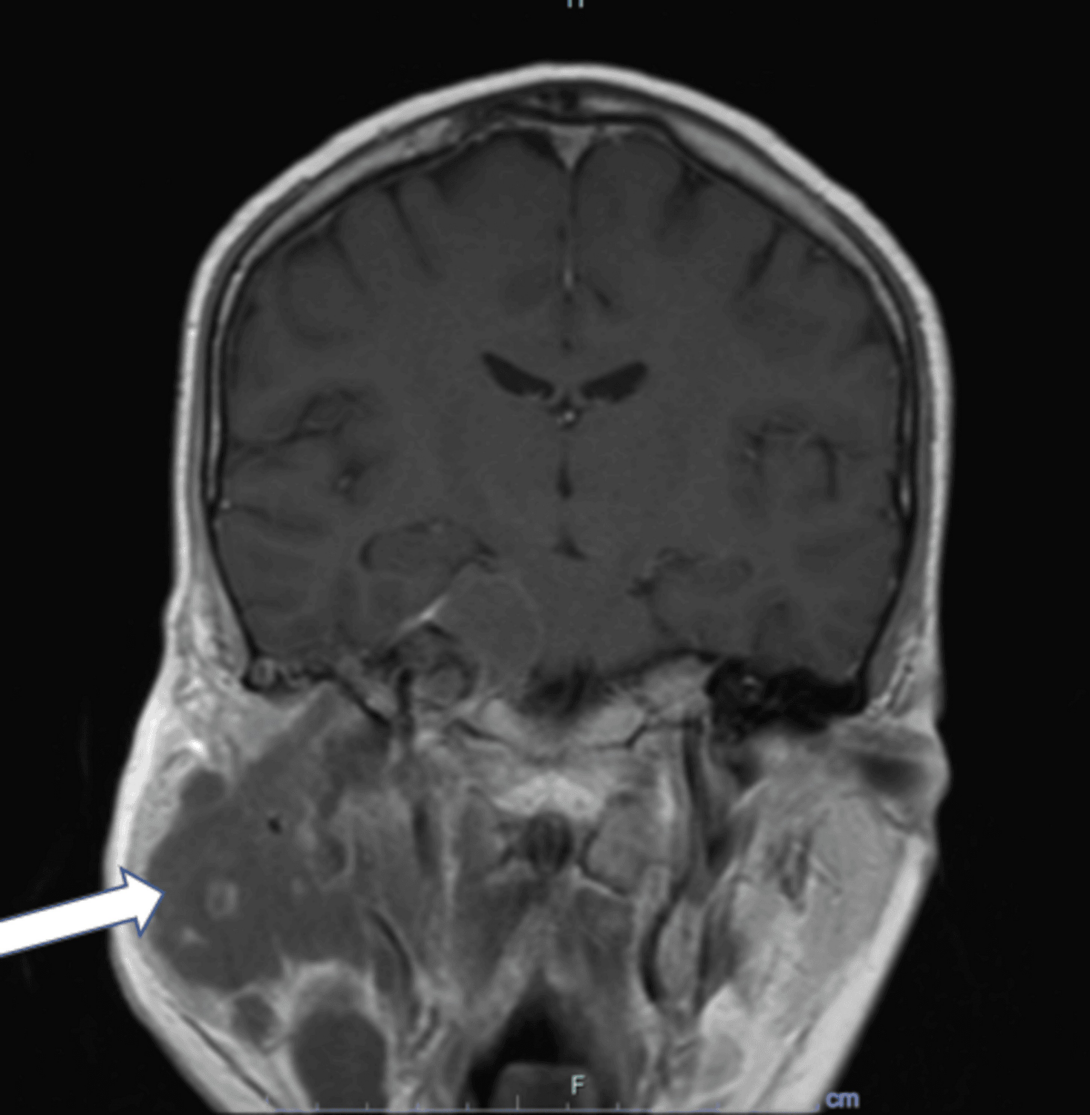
Post-contrast coronal T1-weighted MRI of the brain demonstrating a heterogeneously enhancing mass extending from the right cerebellopontine angle cistern to the right masticator space (white arrow), with extension along the extracranial and intracranial course of the right trigeminal nerve. It measures approximately 84×74 mm in maximal craniocaudal and transverse diameters. A parotid gland biopsy performed shortly after this imaging confirmed the relapse of B-ALL MRI: magnetic resonance imaging; B-ALL: B-cell acute lymphoblastic leukemia
